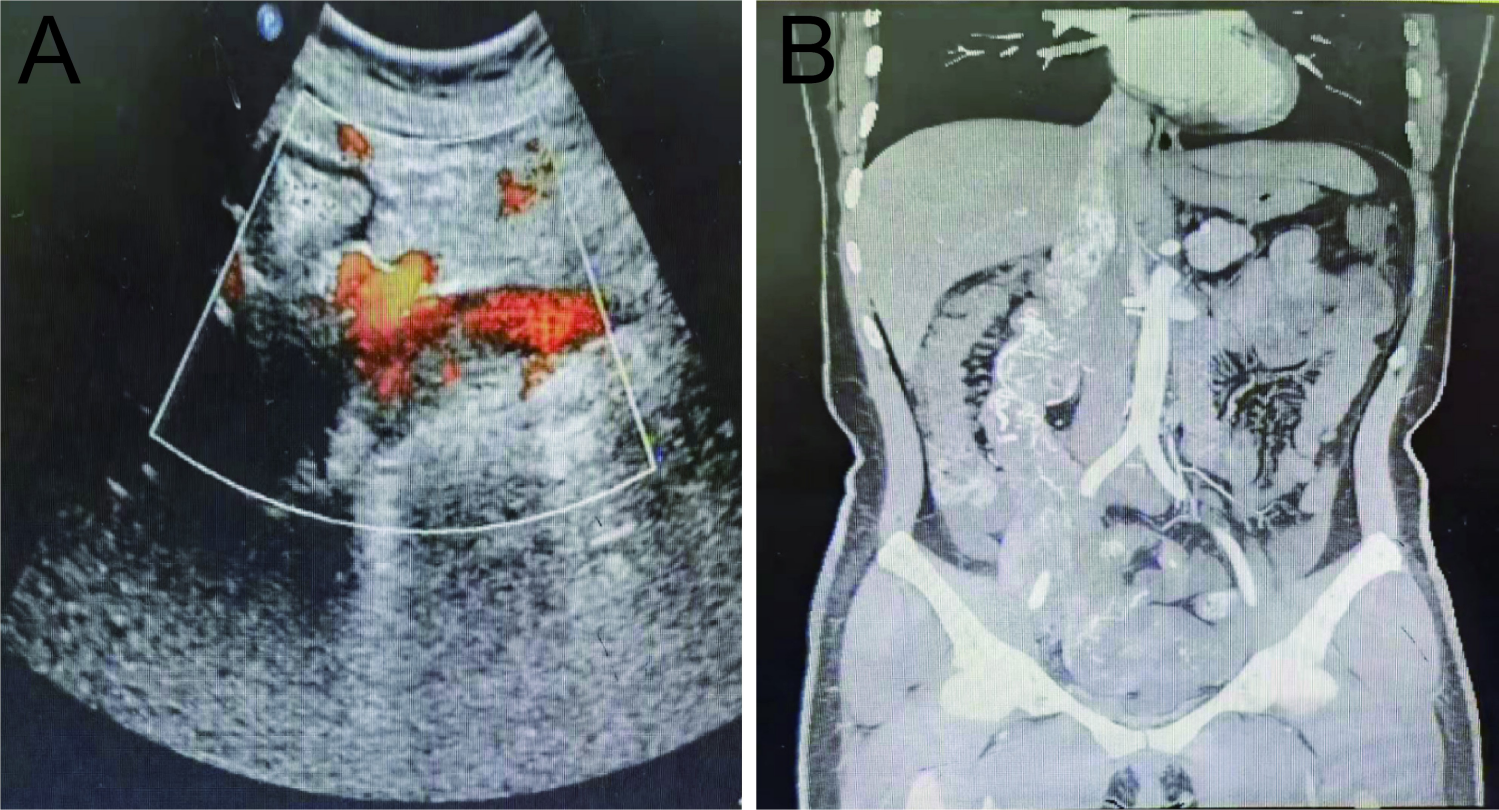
Preoperative pelvic ultrasound and abdominal CTA. (A) Preoperative pelvic ultrasound. (B) Preoperative abdominal CTA.
